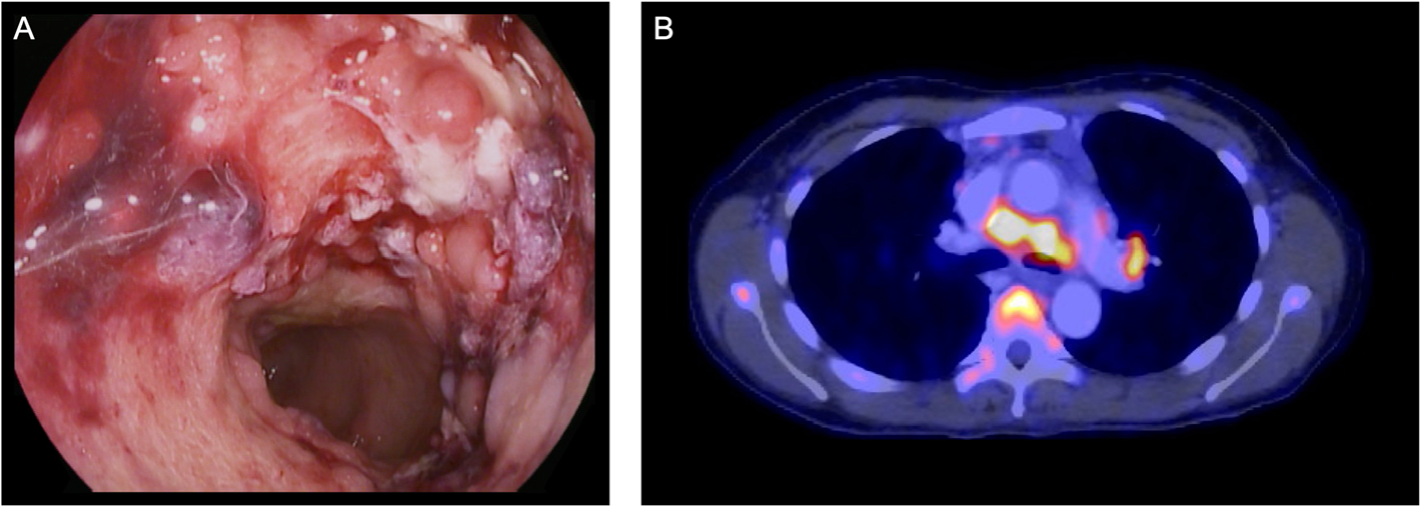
Findings in colonoscopy and PET-CT imaging. a Colonoscopy showing severe, ulcerating colitis due to simultaneous CMV and *Salmonella typhimurium* infections. b Metabolically highly active mediastinal lymphadenopathy in bioptically confirmed disseminated *Mycobacterium intracellulare* lymphadenitis
